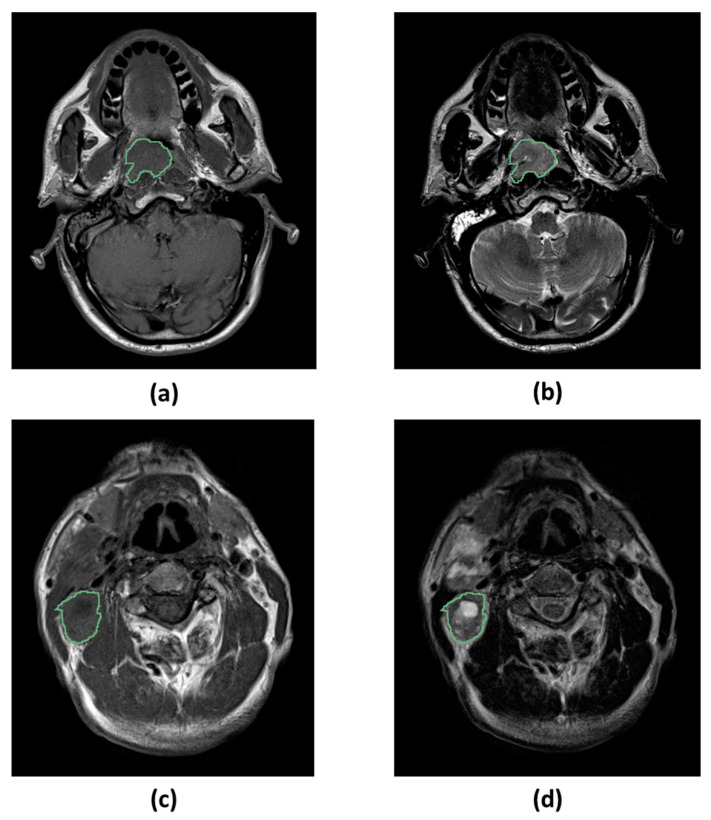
Types of magnetic resonance images and regions of interest used for the study. (a) Primary tumor on T1-weighted image. (b) Primary tumor on T2-weighted image. (c) Largest affected lymph node on T1-weighted image. (d) Largest affected lymph node on T2-weighted image. All the images represent 2D slices from a 3D region of interest.
